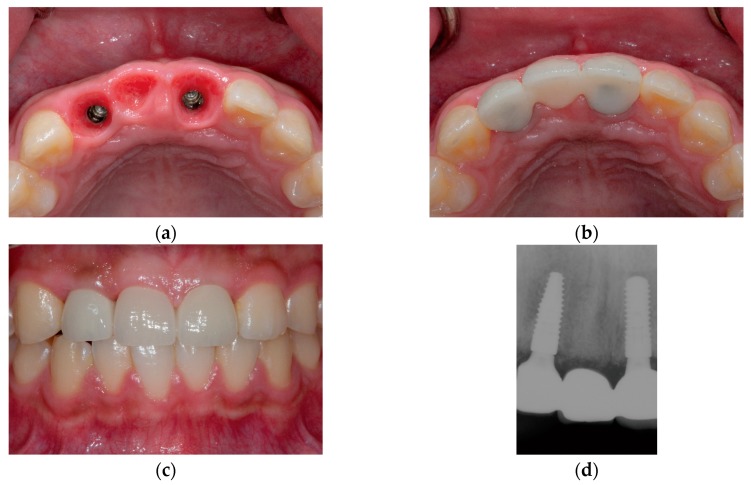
Clinical photographs and a periapical radiograph of the definitive porcelain-veneered zirconia fixed dental prosthesis; (a) Occlusal view after removal of the provisional restoration, showing healthy mucosa profiles; (b) Occlusal view after placement of the definitive restoration; (c) Frontal view after placement of the definitive restoration; (d) Periapical radiograph.
